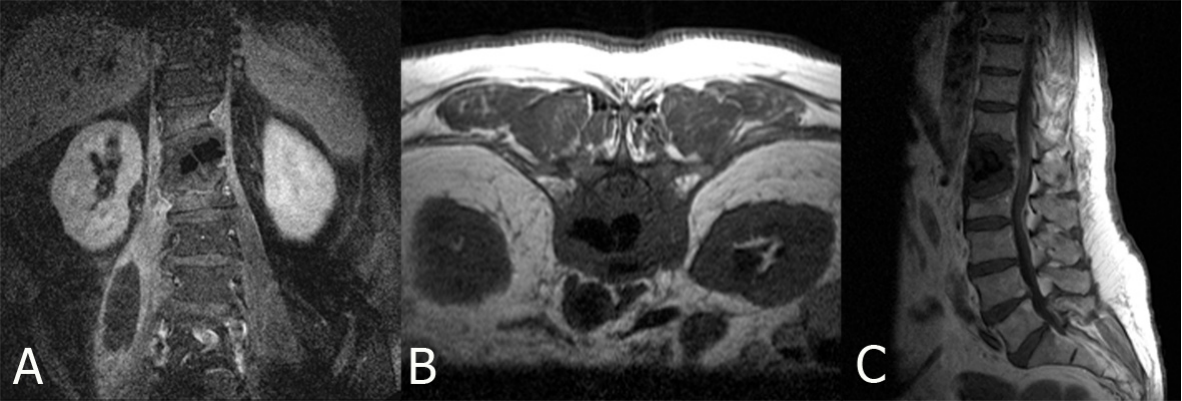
The magnetic resonance imaging T1 gadolinium-enhanced coronal image (A) shows spondylitis and a right-sided psoas abscess. T1 without contrast transverse image of L1 (B) demonstrates the compressed spinal canal and inflamed right psoas muscle. T1 sagittal image (C) shows spinal cord compression.
